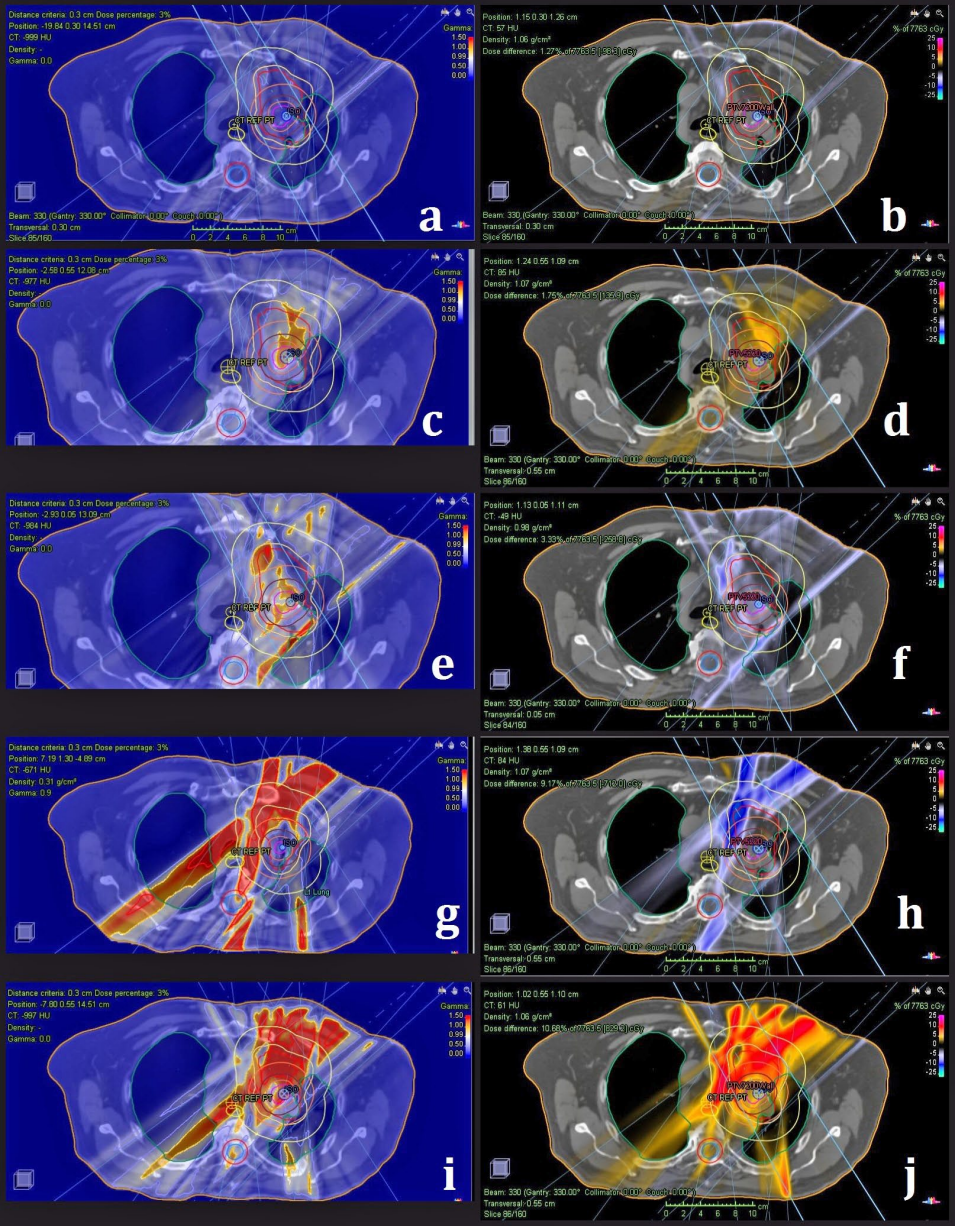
Clinical errors used in this study. Subfigures (a) without introduced error, (c) for 3% MU increase, (e) for 3% MU decrease, (g) for single MLC leaf inserted into the field, (i) for single MLC leaf retracted from the field, show gamma test fail in red overlaid with patient CT for the first thoracic case. Subfigures (b), (d), (f), (h), (j) show TPS computed and COMPASS measured absolute dose difference for the same errors and the same patient.
